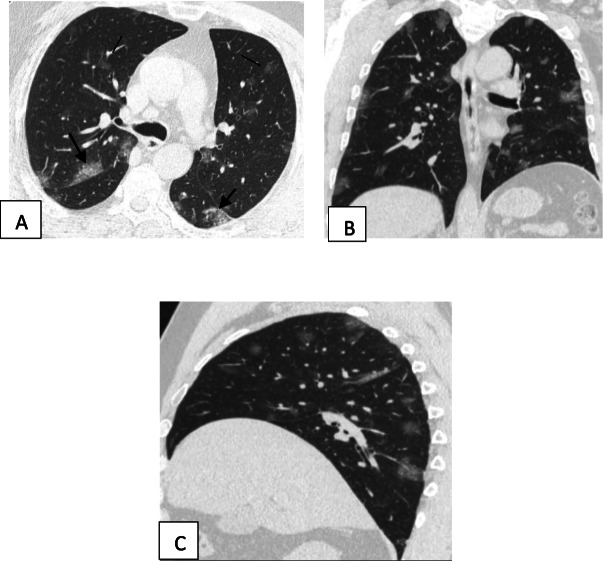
Unenhanced chest CT of a 52-year-old male patient with COVID-19. a An axial lung window image shows bilateral subpleural and broncho-vascular areas of ground glassing opacity [lines] and crazy paving [arrows]. b Coronal and c sagittal reformatted images. CT score is 12
